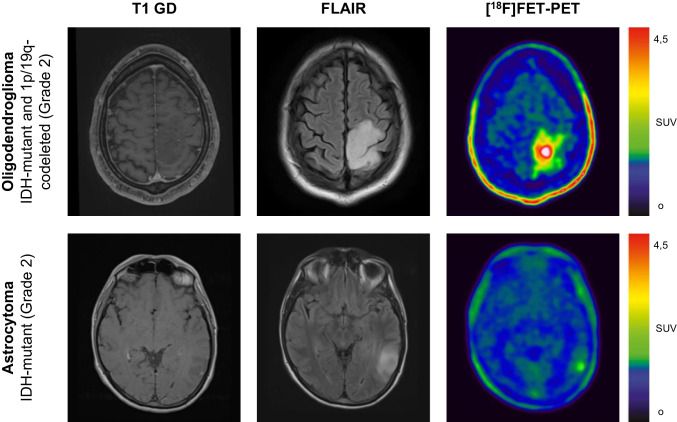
[^18^F]FET PET and MRI of different grade 2 diffuse gliomas. Contrast enhanced T1-weighted MRI, FLAIR and [^18^F]FET image fusion of patients with left parietal oligodendroglioma (*top row*) and left temporal astrocytoma (*bottom row*)
